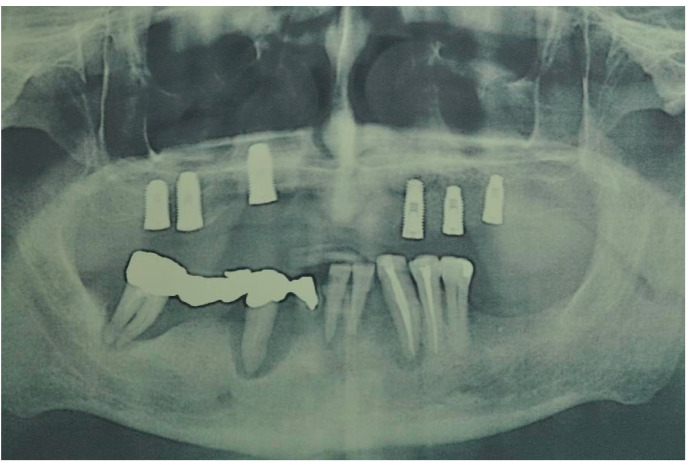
Postoperative orthopantomography in the upper arch.
